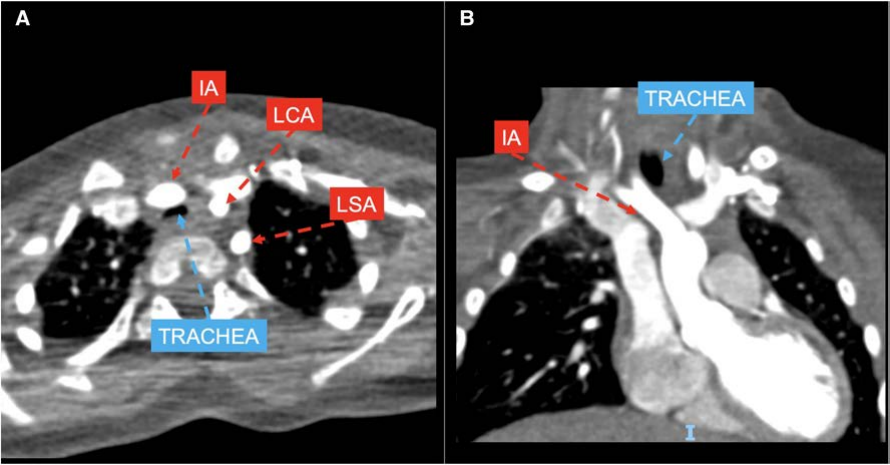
CT axial (A) and coronal (B) views showing left aortic arch with normal branching pattern and innominate artery (IA) anteriorly and laterally compressing the trachea; LCA, left coronary artery; LSA, left subclavian artery.
